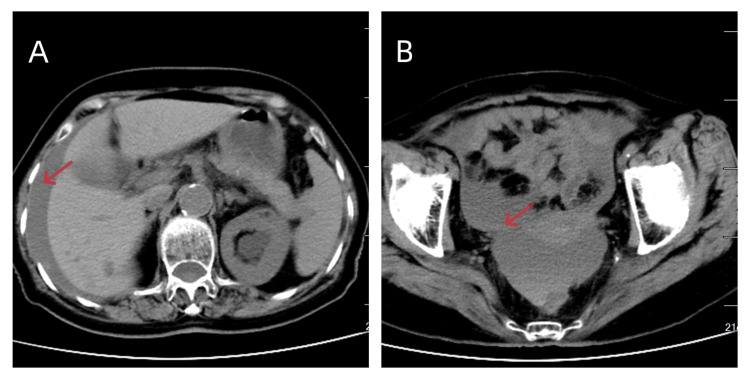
CT scan showed seroperitoneum in the perihepatic space (A) and pelvic cavity (B). No free air was noticed.
